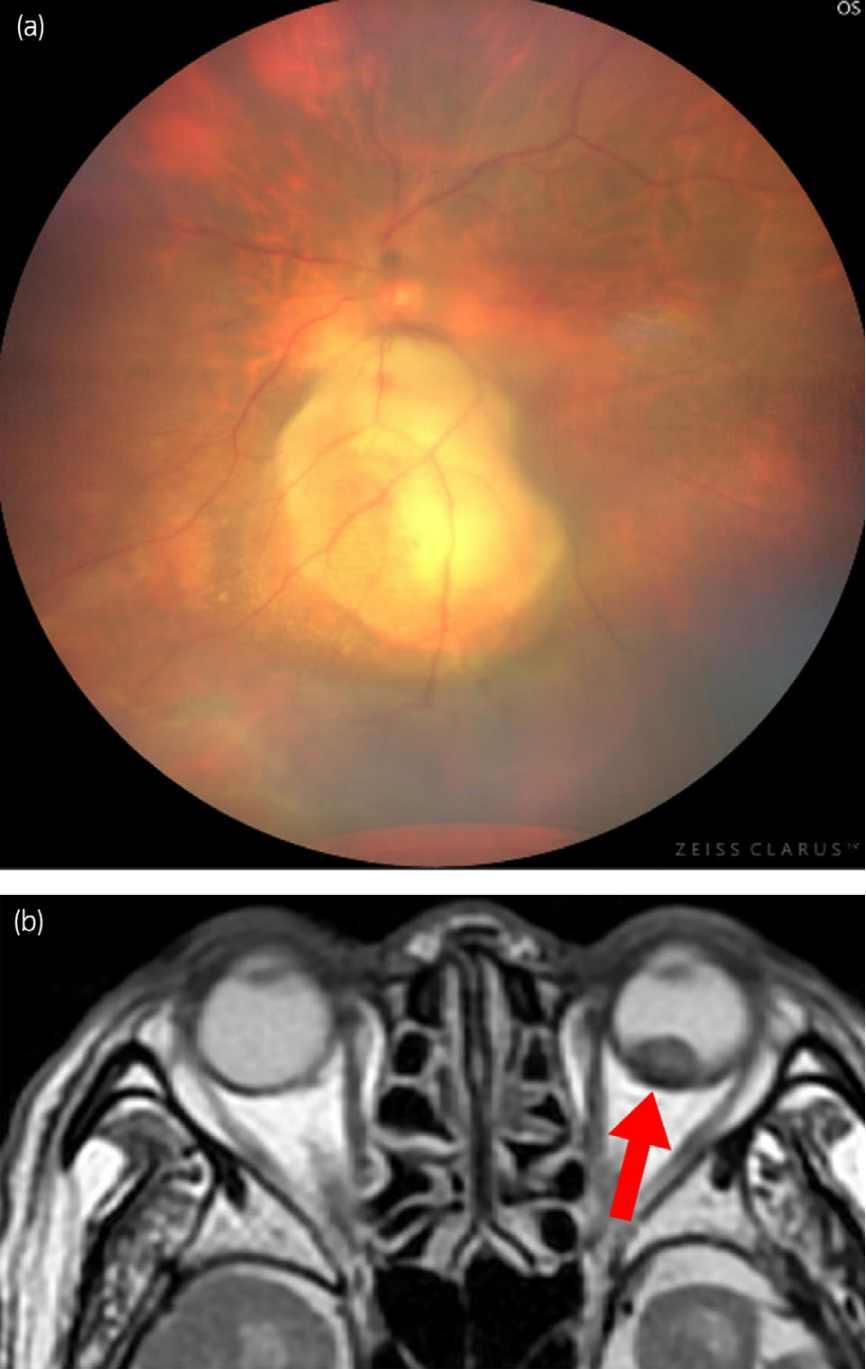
(a) Fundus examination revealing serous retinal detachment and a tumor in the choroid. (b) T2‐weighted axial magnetic resonance imaging (MRI) revealing a hypointense mass within the left eye measuring 10 mm in diameter.
